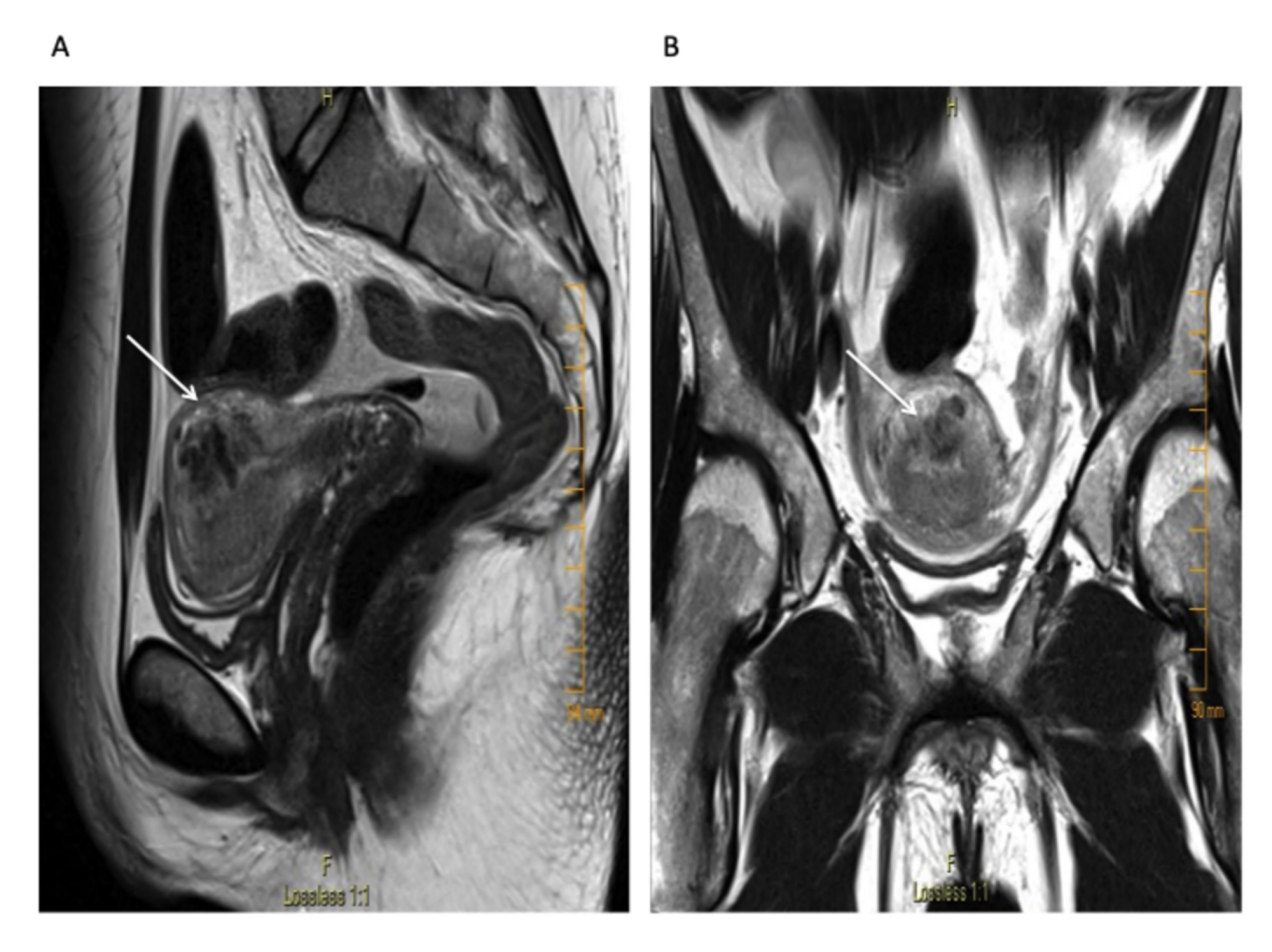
T2-weighted pelvic magnetic resonance imaging (MRI) revealing a hypointense T2 lesion within the posterosuperior wall of the myometrium: (A) sagittal view (B) axial view
